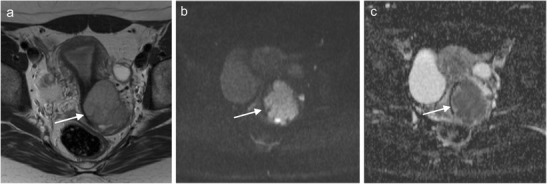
Axial T2-weighted image (a) and DWI image (*b* = 800) with ADC map (c) of a 36-year-old woman with stage IIB low-grade squamous cell cervical carcinoma. A hyperintense lesion is seen in the cervix (*arrow*), and there is disruption of the cervical stroma ring and parametrical invasion (a). The tumour shows high signal intensity on DWI (b), with corresponding low signal intensity on the ADC map (c)
